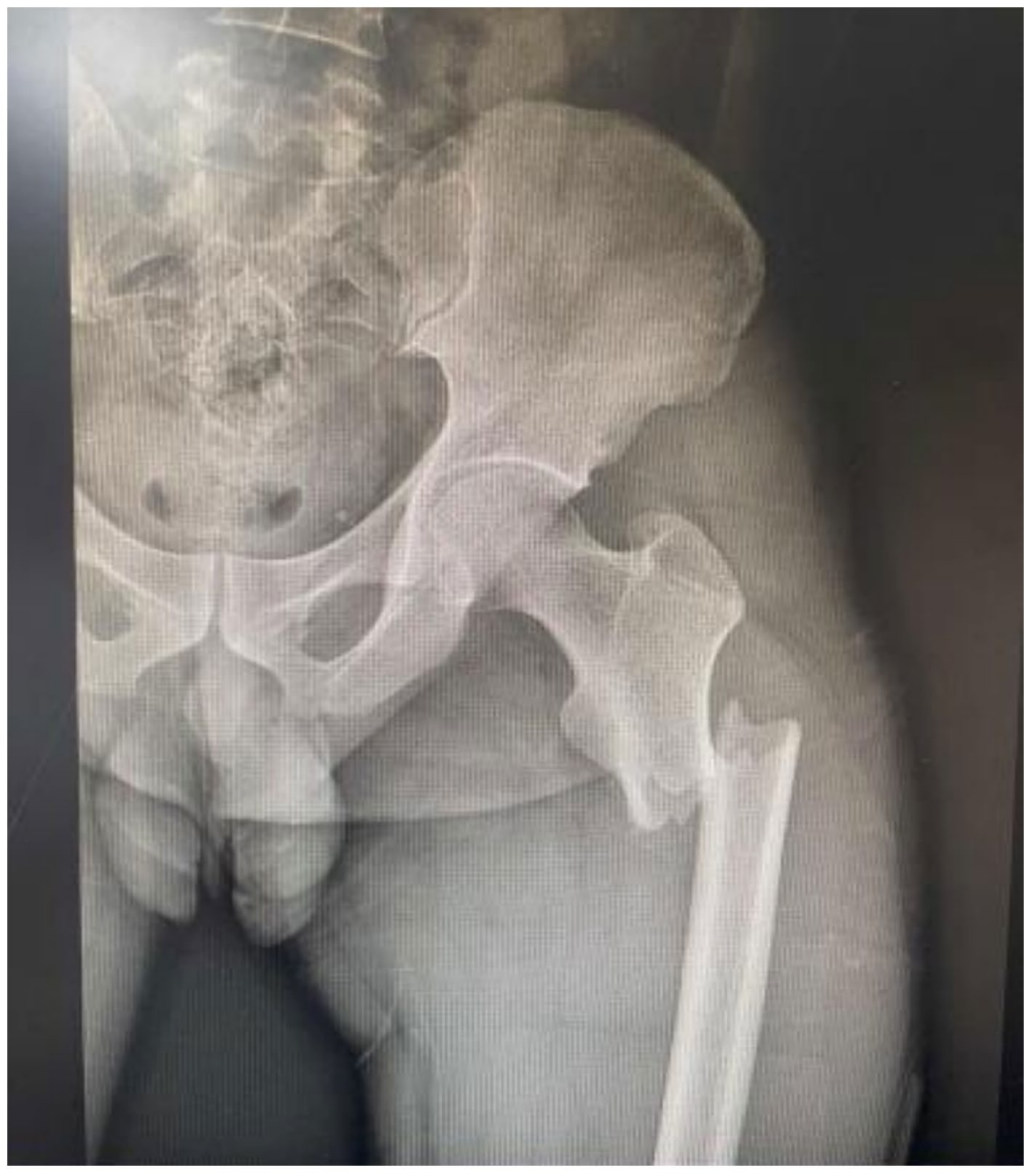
X-ray film of femoral fracture of the patient.
